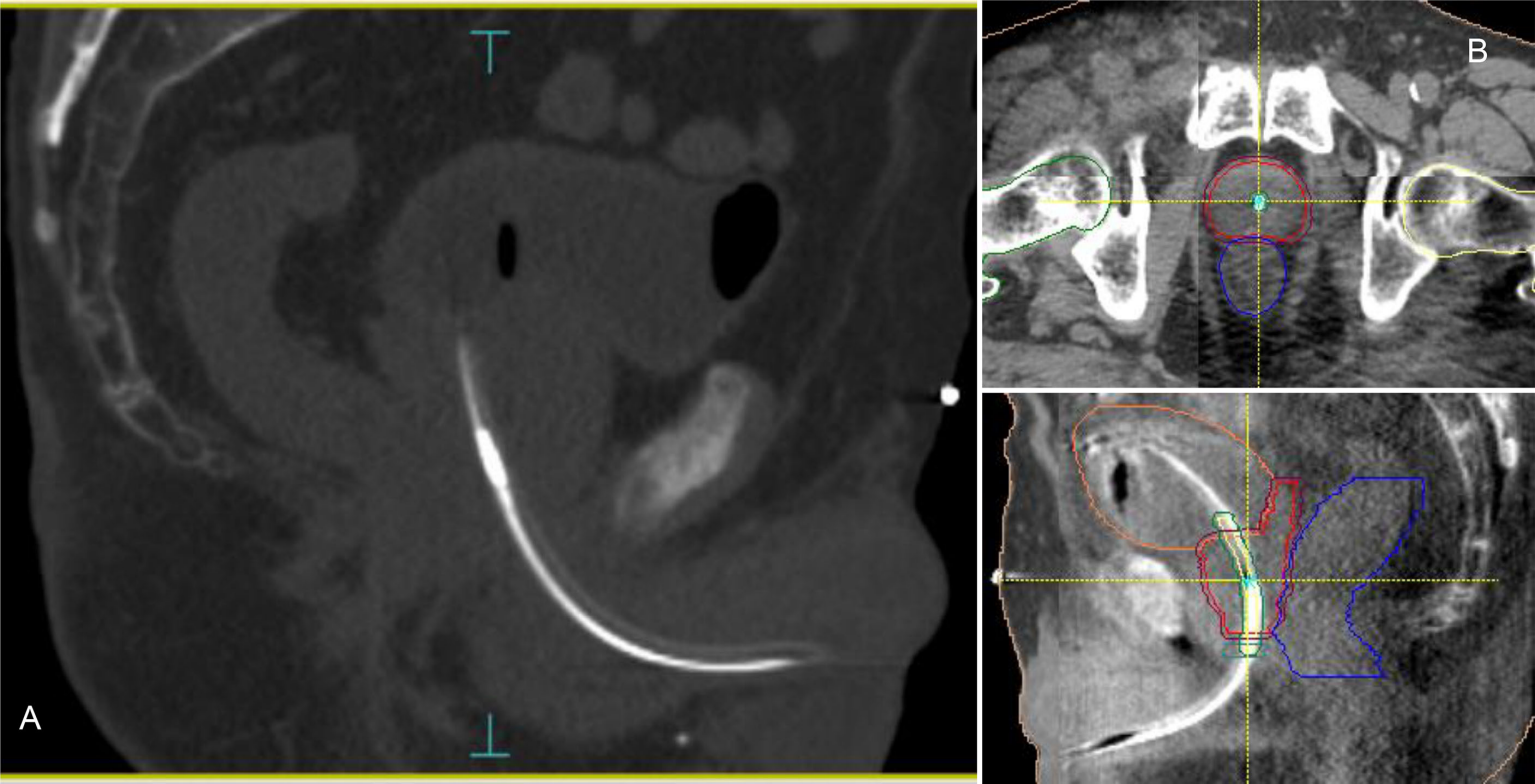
RayPilot HypoCath. The transmitter choke is visible inside the urinary catheter within the prostatic urethra (A). Planning CT to daily CBCT matching: proper rectum and bladder filling verification, in addition to transmitter and urethra localizations; in case of deformation or deviation of the urethral path, the catheter was placed inside the urethra PRV along with the entire extension of the prostate (B).
